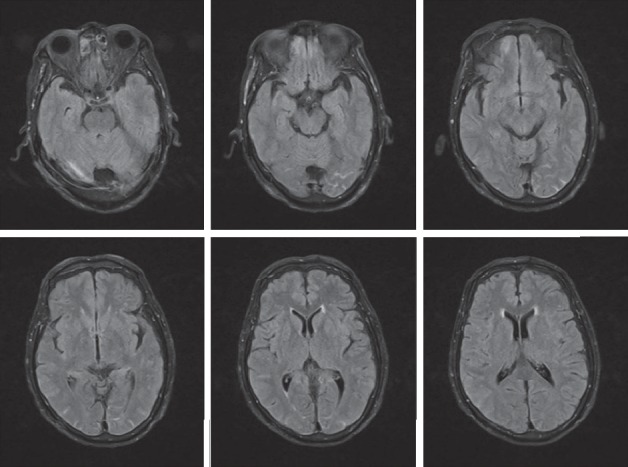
The MRI image in July 31st. A small amount of bleeding was displayed in the occipital lobe.
